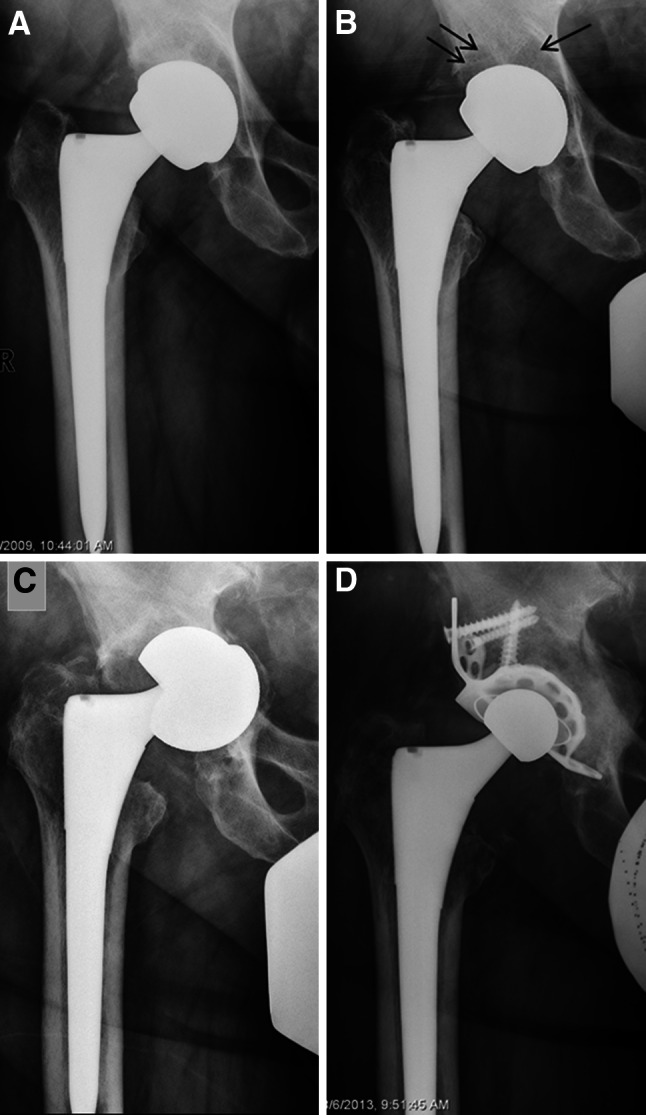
Standardized anteroposterior (AP) pelvis radiographs showing a Birmingham modular metal-on-metal total hip arthroplasty in a 65-year-old man (patient 2): a well-aligned components were observed 8 weeks after surgery; b periprosthetic lucencies in DeLee zone 1 and 2 were evident 4 months prior to revision surgery (*black arrows*); c the loose right cup was seen on the day of revision surgery and d AP pelvis radiograph 8 weeks after revision surgery demonstrating high-density bone grafting and an acetabular cage with a cemented liner
